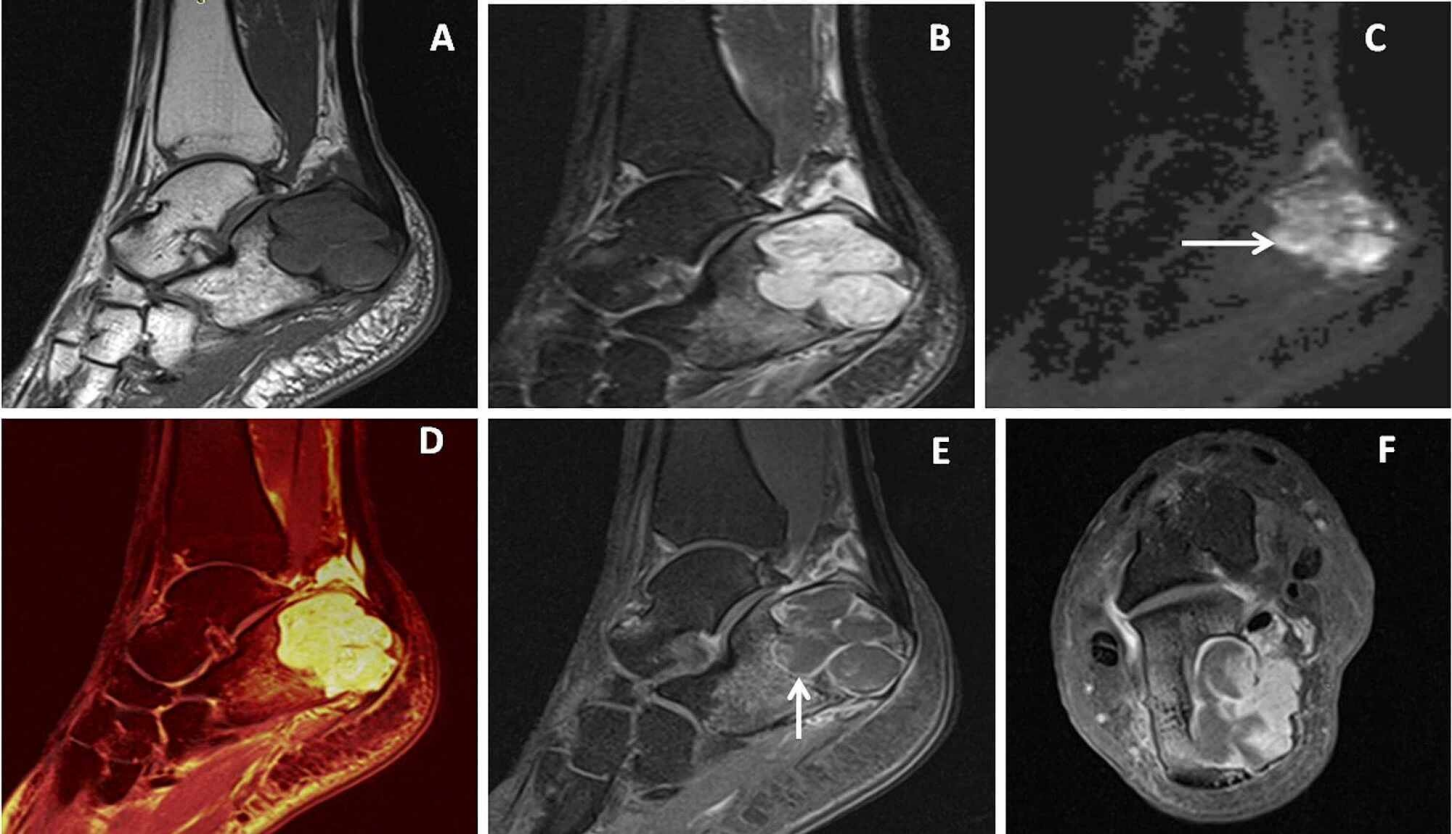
Calcaneal giant cell tumor (GCT) Thirty-six-year-old female with calcaneal GCT. Sagittal T1 weighted image (T1WI) and proton density fat-suppressed (PDFS) images (A &B) showed T1WI hypo and PDFS hyperintense expansile lesion in the posterior portion of calcaneum. Sagittal diffusion-weighted imaging (DWI) (C) showed diffusion restriction (→ arrow), where fusion image (D) between sagittal PDFS and DWI showed bright signals within the lesion. Post gadolinium fat-suppressed sagittal and axial T1W images (E &F) showed moderate heterogeneous enhancement of the mass with more irregular peripherally enhancing solid components with enhancing septae (↑ arrow ).
